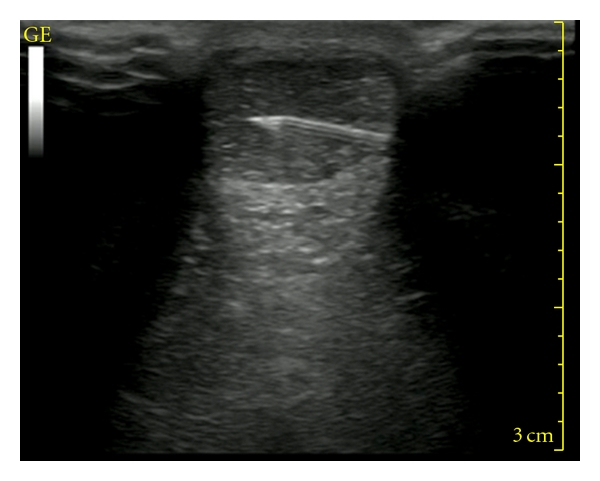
Ultrasonographically guided intratendinous Achilles injection.
